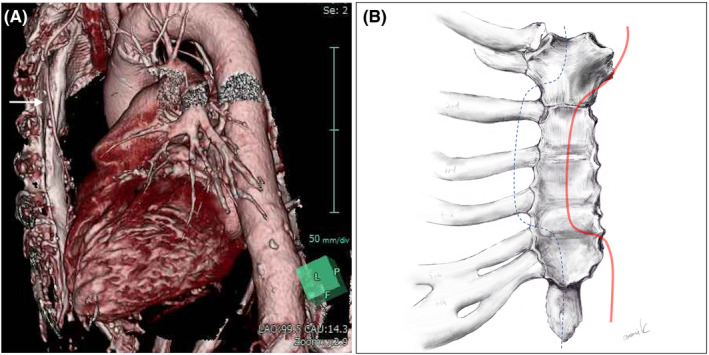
A, Preoperative three‐dimensional enhanced computed tomography revealed a patent left intrathoracic artery graft just below the sternum (white arrow); (B) depiction of modified median sternotomy with dissection of the second, third, and fourth right costal cartilages (blue line: sternotomy line, red line: left intrathoracic artery graft)
